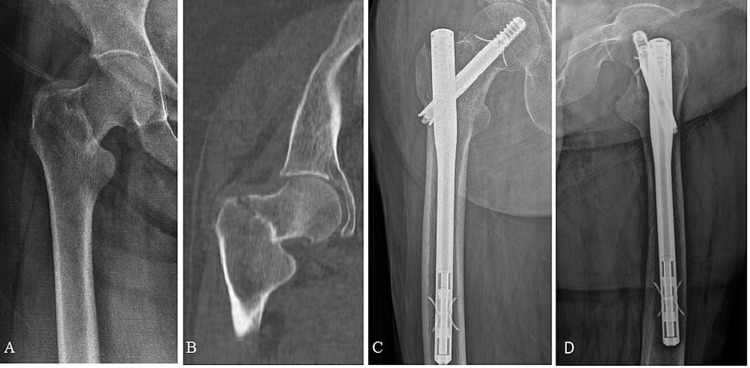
(A) Radiograph of a two-part basicervical fracture in a 91-year-old female. (B) A computed tomography image shows the coronal section of the fracture level. (C, D) Postoperative anteroposterior and lateral images at six months show no telescoping.
